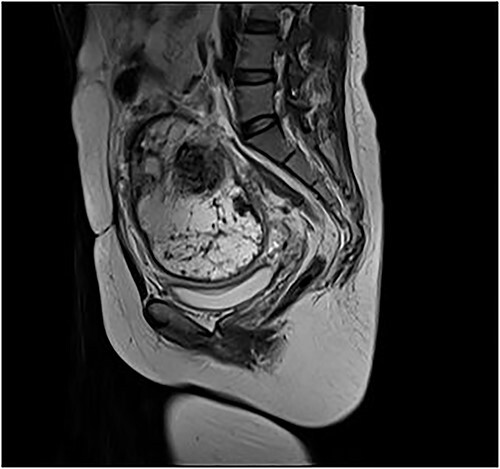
Pelvic enhancement MRI showing large intra-abdominal mass.
